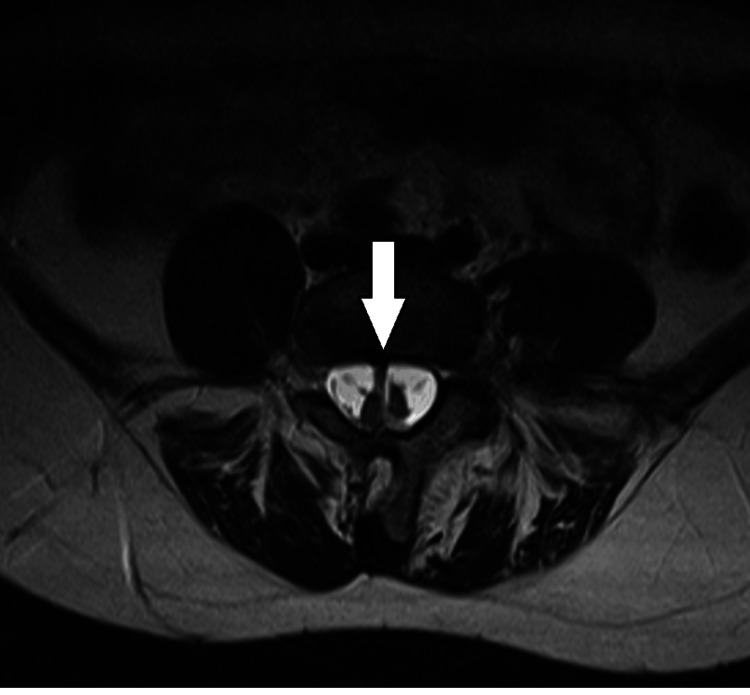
MRI of L4-L5 diastematomyelia (axial plane) The hemicords have been split by a bony spur. The arrow points at the bony spur between the hemicords.
